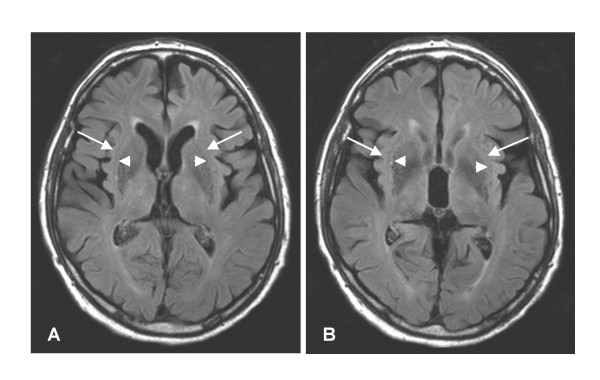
Axial T2 FLAIR images along the course of the IFO. MRI T2 FLAIR images, in a patient with PSP and IFO atrophy. The figures show adjacent axial sections (A, B), which include the external capsule (short arrows) and extreme capsule (long arrows). The IFO passes through both these structures. In each section a single minimal white matter signal intensity can be seen in the right external capsule. Note the absence of signal changes in white matter in general, in spite of the presence of mild frontal cortical atrophy.
